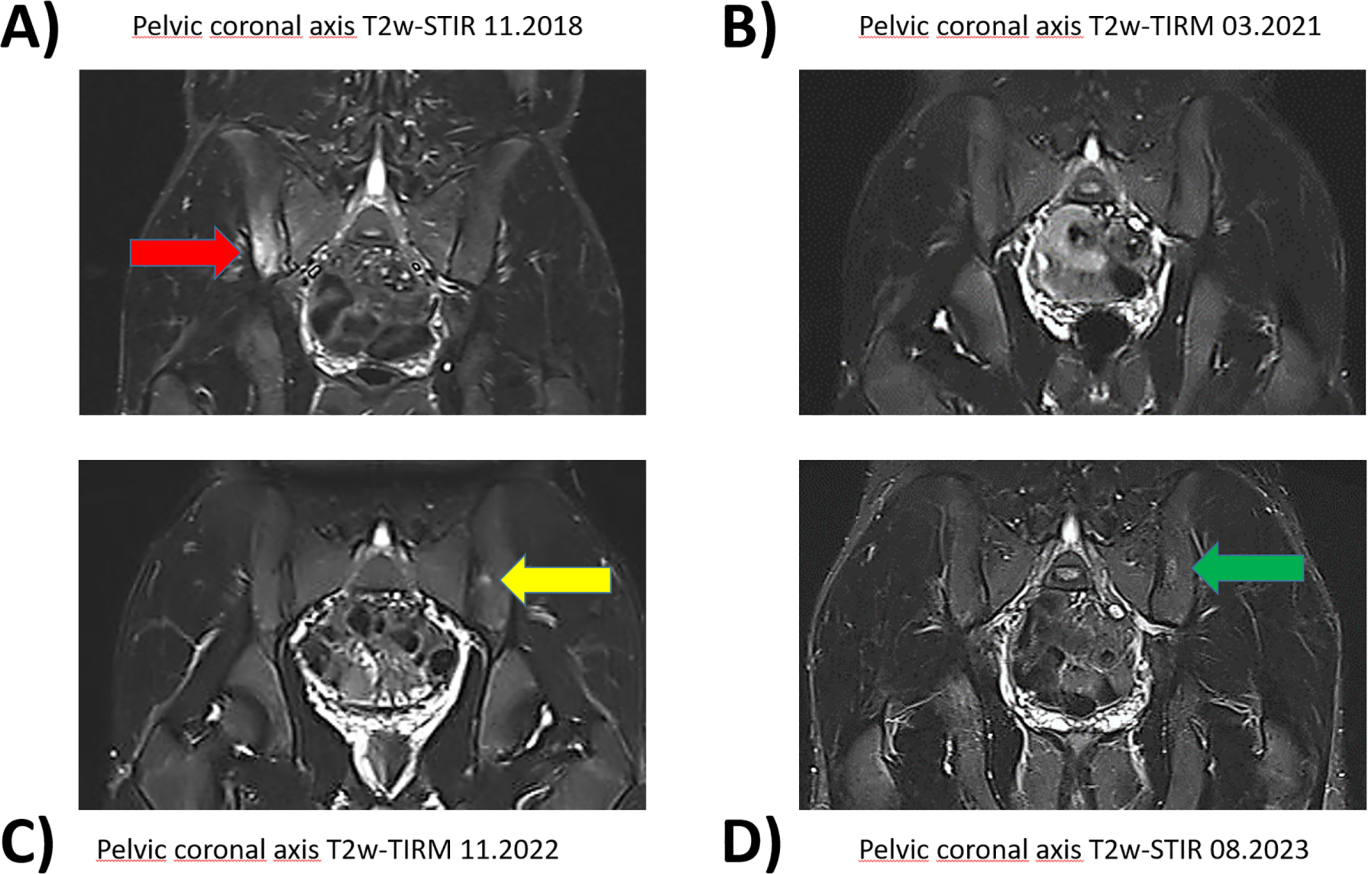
Pelvic magnetic resonance imaging with fat suppressed sequences. Corresponding pelvic coronary planes in MRI imaging during the course of the disease between 11.2018 and 08.2023. The fat suppressed sequences T2-weighted short-tau inversion recovery (T2w-STIR) and T2-weighted turbo inversion recovery magnitude (T2w-TIRM) were used for image acquire. (A) Hyperintense edema signal representing osteitis was found in the right iliac bone and adjacent lateral mass in 11.2018(red arrow). (B) Adalimumab treatment led to a good response with regression of edema. (C) New lesion on the left side after discontinuation of adalimumab 05.2022 (yellow arrow). (D) Persisting inflammation despite initiation of secukinumab in 11.2022 (green arrow).
